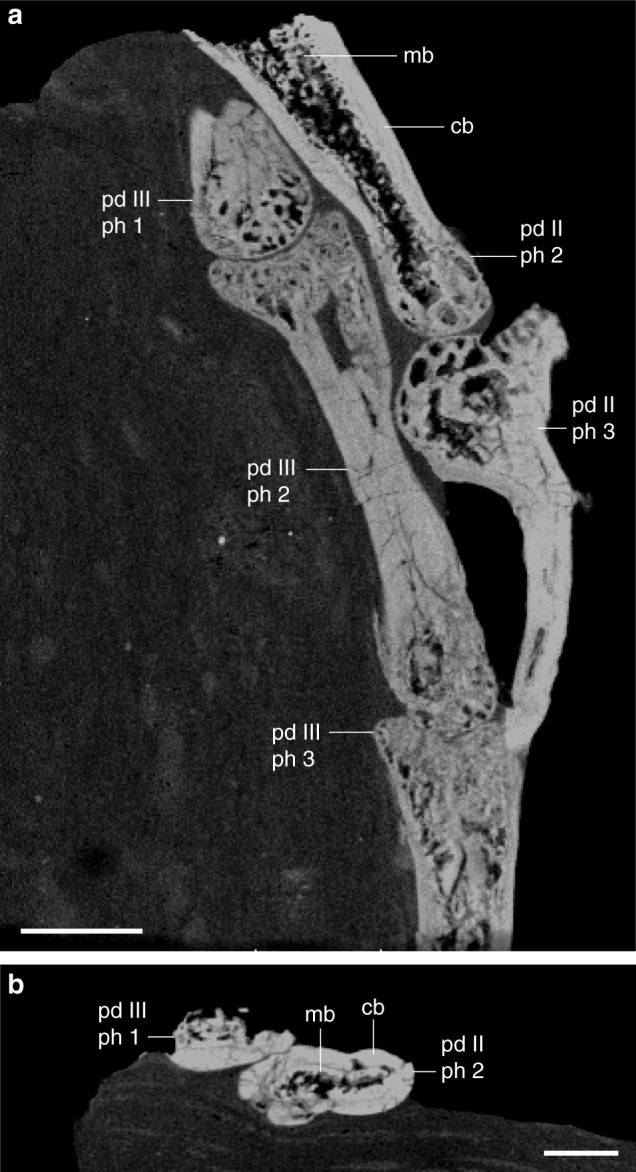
CT-scans of the distal pedal phalanges showing the penultimate phalanx of pedal digit II with small amounts of medullary bone lining the internal cavity. a Medial slice along the *x*-axis showing pedal digits II and III; b same section, *y*-axis. Scale bar equals 1000 μm. Anatomical abbreviations not listed in Fig. 2 caption: ph, phalanx
